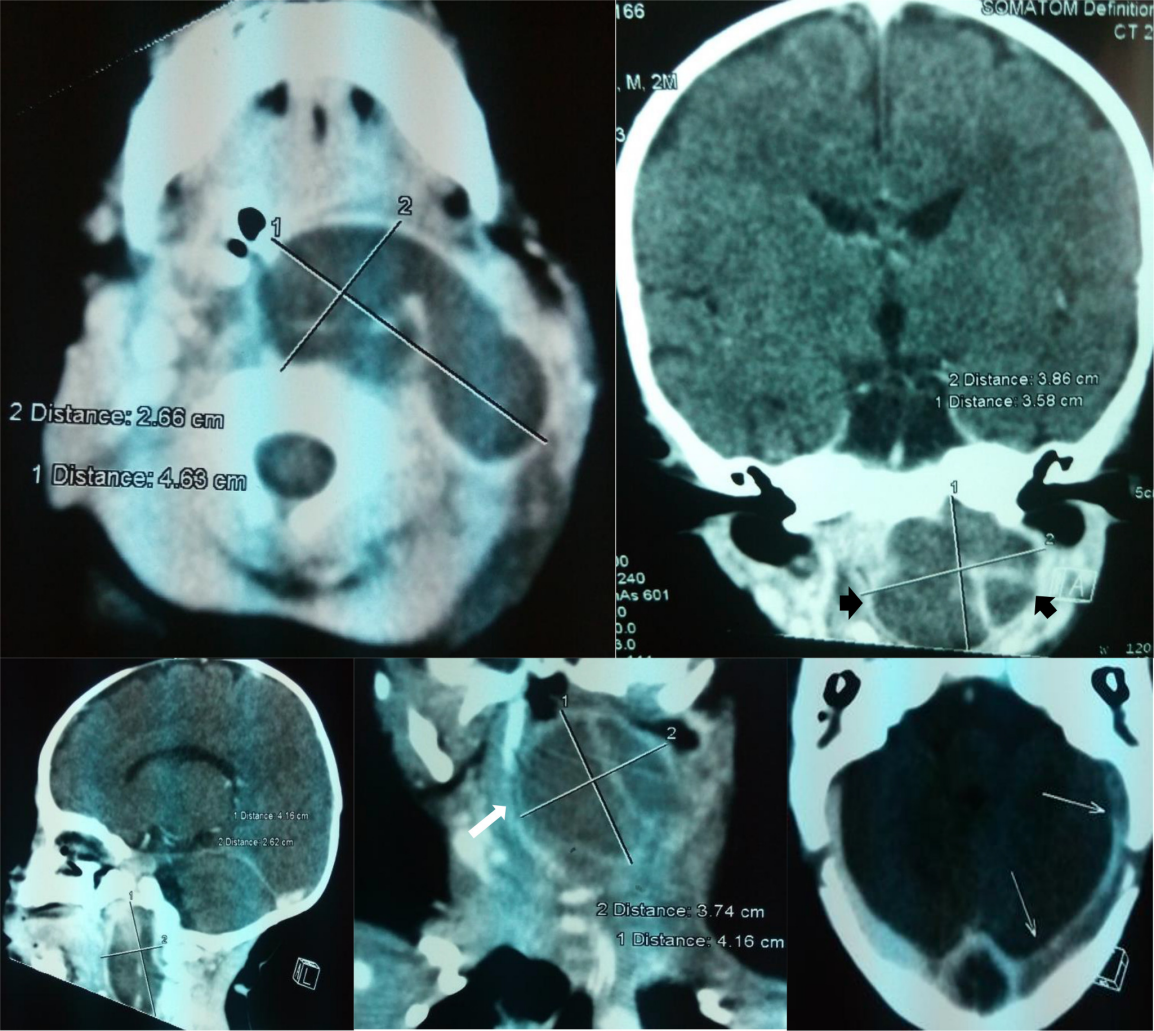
(a–e) Contrast enhanced CT scan images at the level of naso/oropharynx: axial (a), sagittal (c) and coronal (b) and (d) revealing an abscess within right retropharyngeal and parapharyngeal spaces seen as a low density lesion with thin rim of peripheral enhancement (black arrowheads on b). Also note the massive displacement of the trachea ((white arrow in d) and lateral sinus thrombosis on e) seen as filling defects in the sinus (white arrows).
